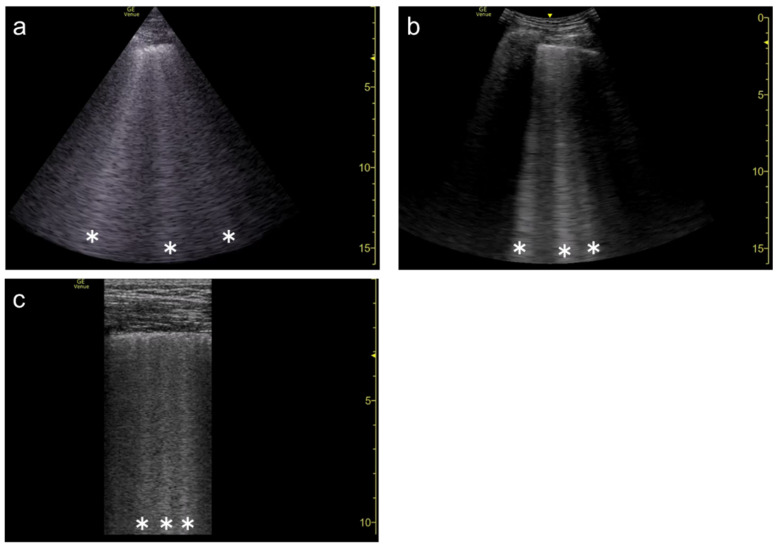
Ultrasound images obtained with Venue scanner (GE Healthcare) with a sector (a), curvilinear (b), and linear (c) transducers in cardiogenic pulmonary edema. Asterisks indicate B-lines.
